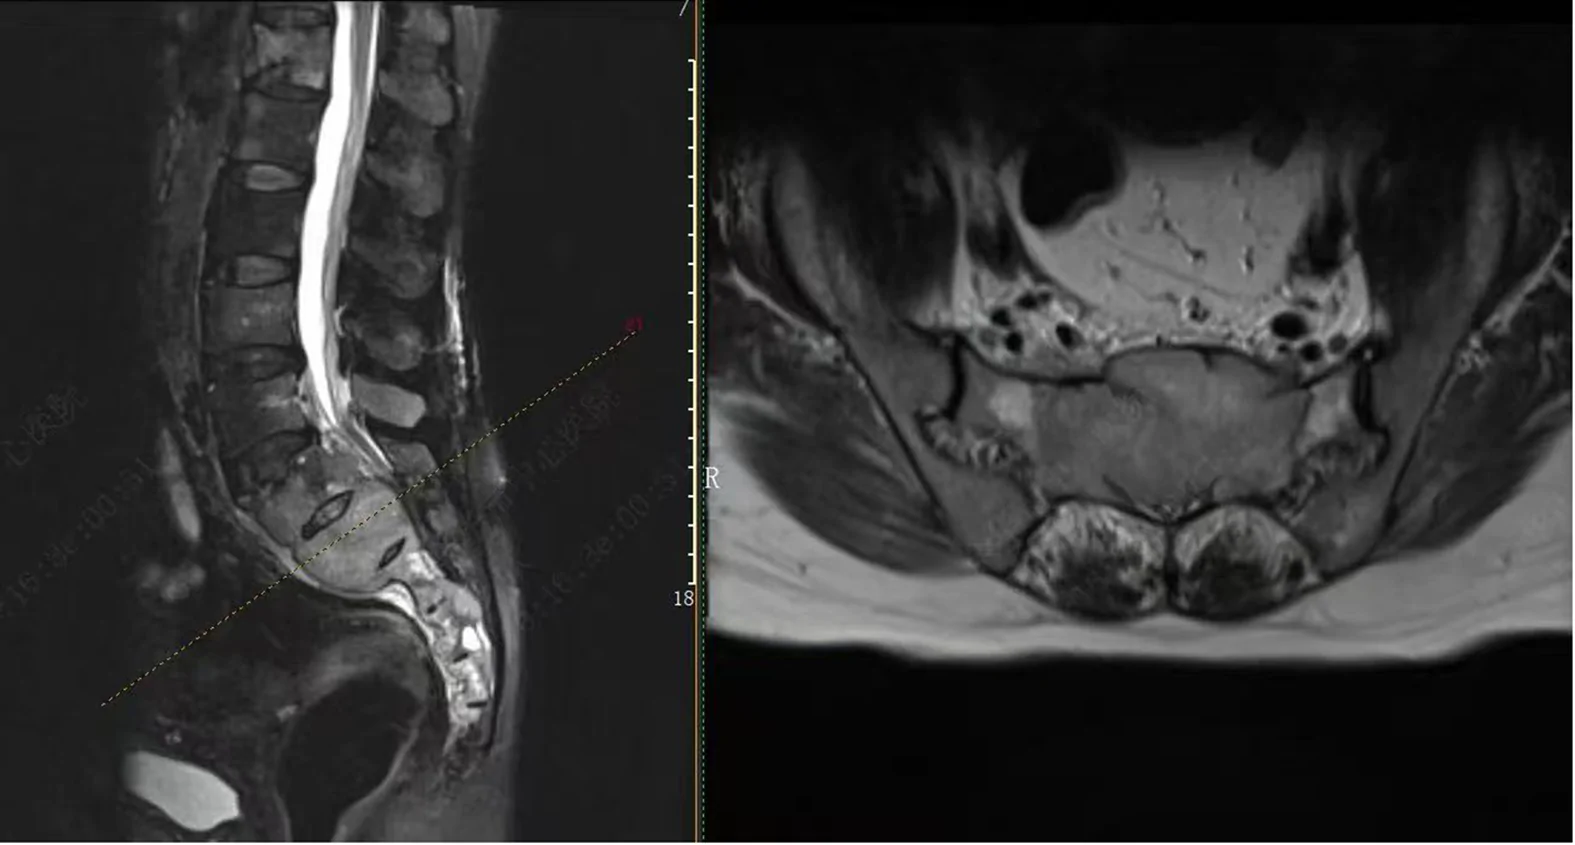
Spinal MRI of the patient demonstrated multiple patchy ill-defined abnormal signals in the thoracic, lumbar and sacrococcygeal vertebrae, consistent with bone metastasis.
